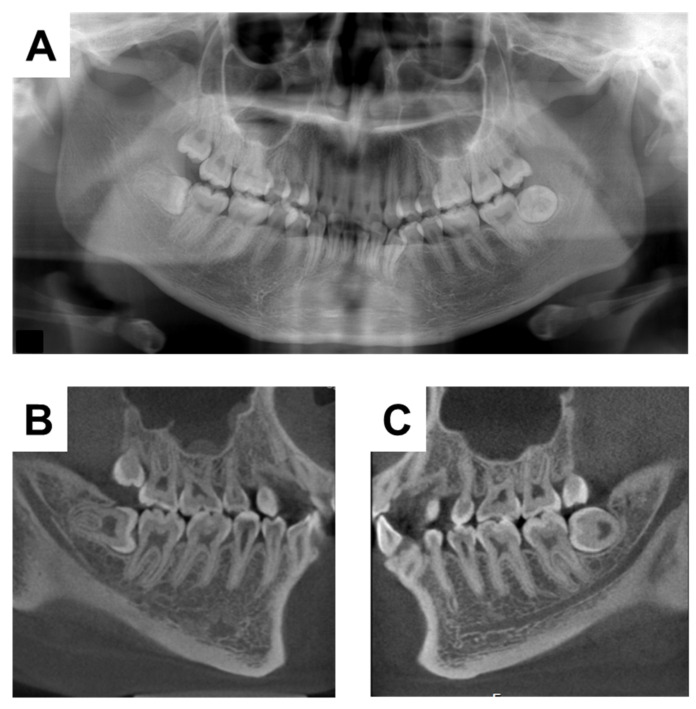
X-ray examinations at the initial visit. (A) Orthopantomograph. (B) Computerized tomographic image of the right side. (C) Computerized tomographic image of the left side.
